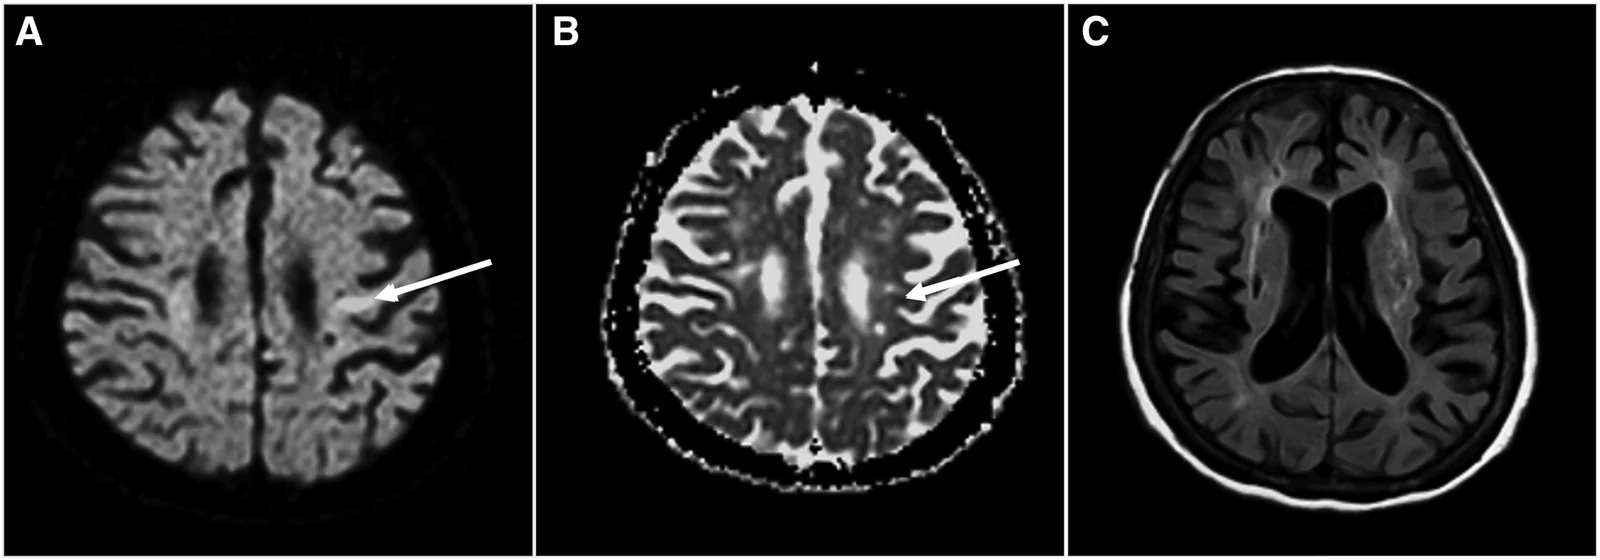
Magnetic resonance imaging of the brain demonstrating a small acute infarction in the left corona radiata in the setting of advanced cerebral small vessel disease. (A) Diffusion-weighted imaging demonstrates a small focal area of restricted diffusion in the left corona radiata (arrow), consistent with an acute lacunar infarction. (B) The corresponding apparent diffusion coefficient map demonstrates reduced signal intensity at the same location (arrow), confirming restricted diffusion. (C) Fluid-attenuated inversion recovery imaging demonstrates extensive periventricular and deep white matter hyperintensities (Fazekas grade 3) together with multiple chronic lacunar infarcts, compatible with advanced cerebral small vessel disease.
